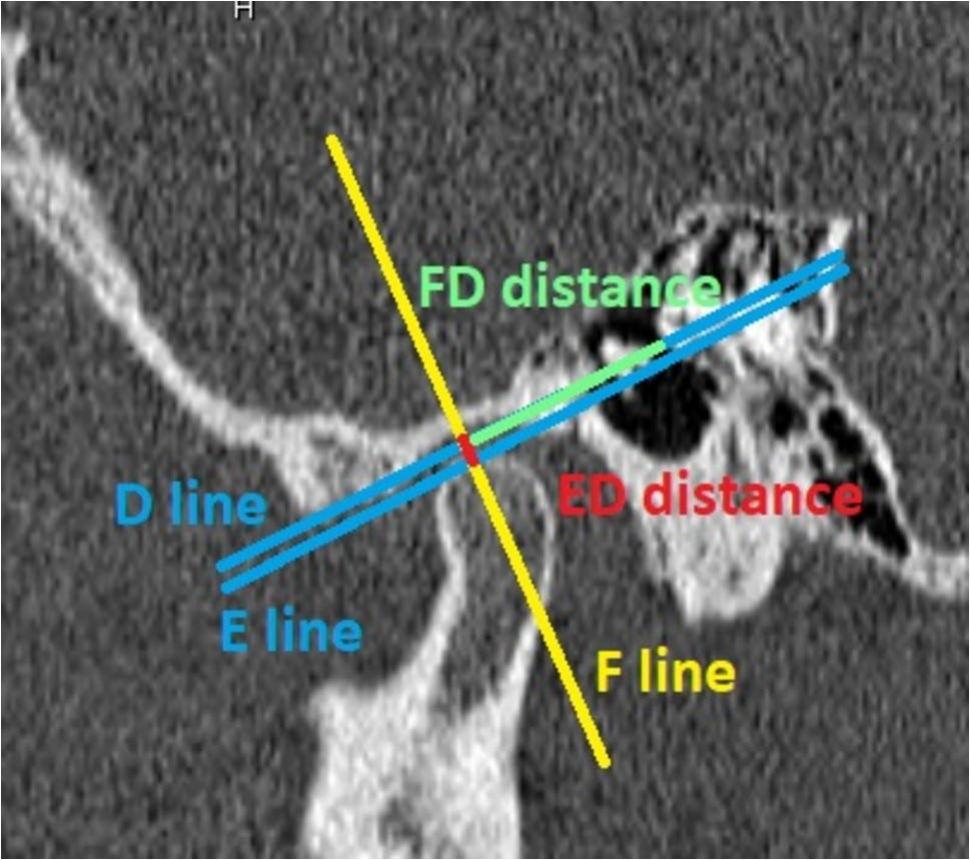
Sagittal CT condylar position parameters
